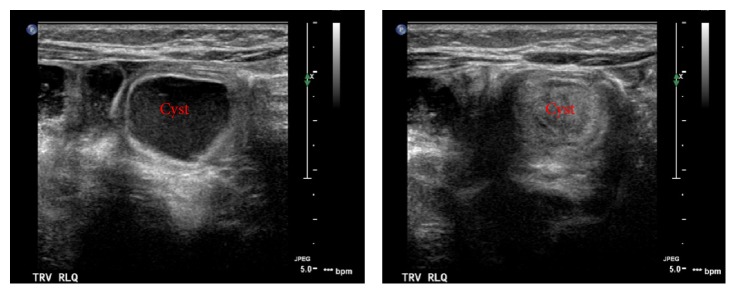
Abdominal ultrasound demonstrating a cystic structure in the right lower quadrant.
